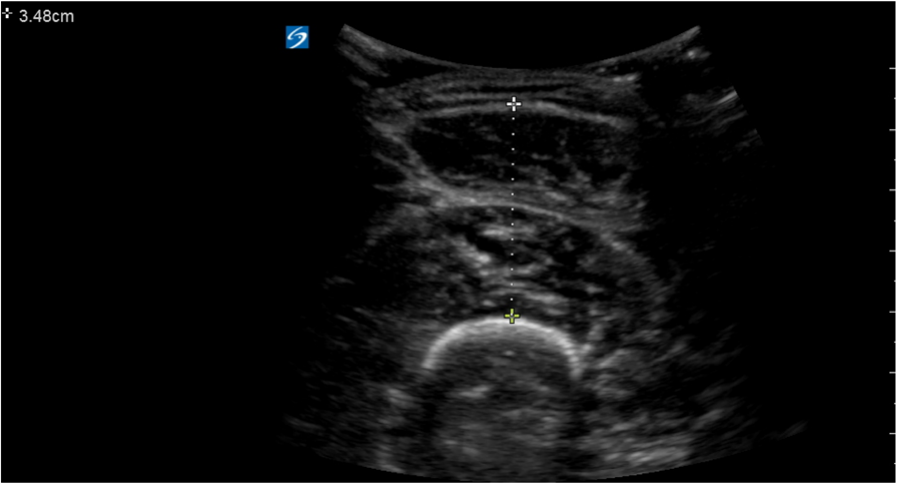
Ultrasound image of the quadriceps, measured at mid thigh. Calipers demarcate the muscle thickness, measured from the perimysium of the rectus femoris to the cortex of the femur
